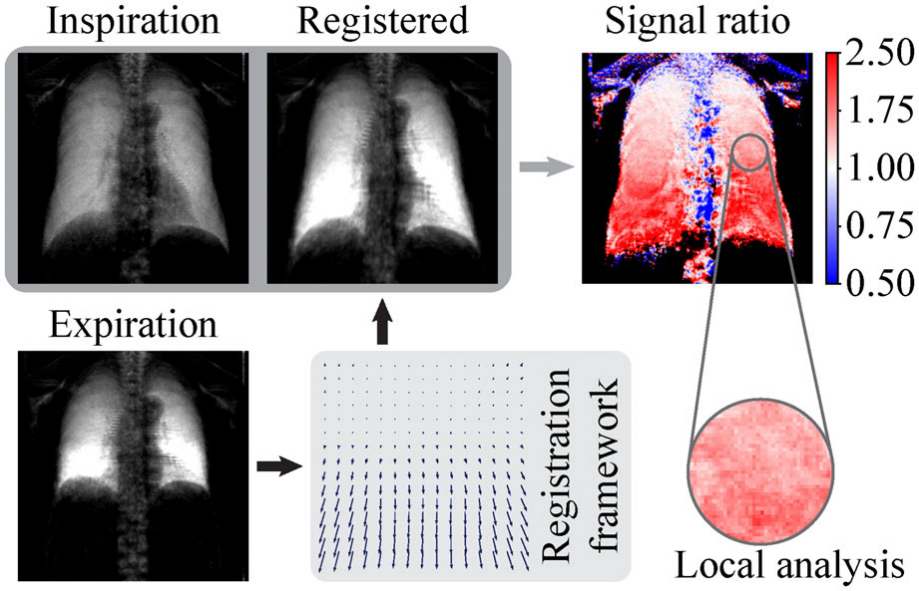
Dark‐field‐compatible deformable image registration methods enable matching chest radiographs in full expiration and full inspiration. The ratio of the transformed expiration and inspiration dark‐field images is used to analyze local lung signal changes.
